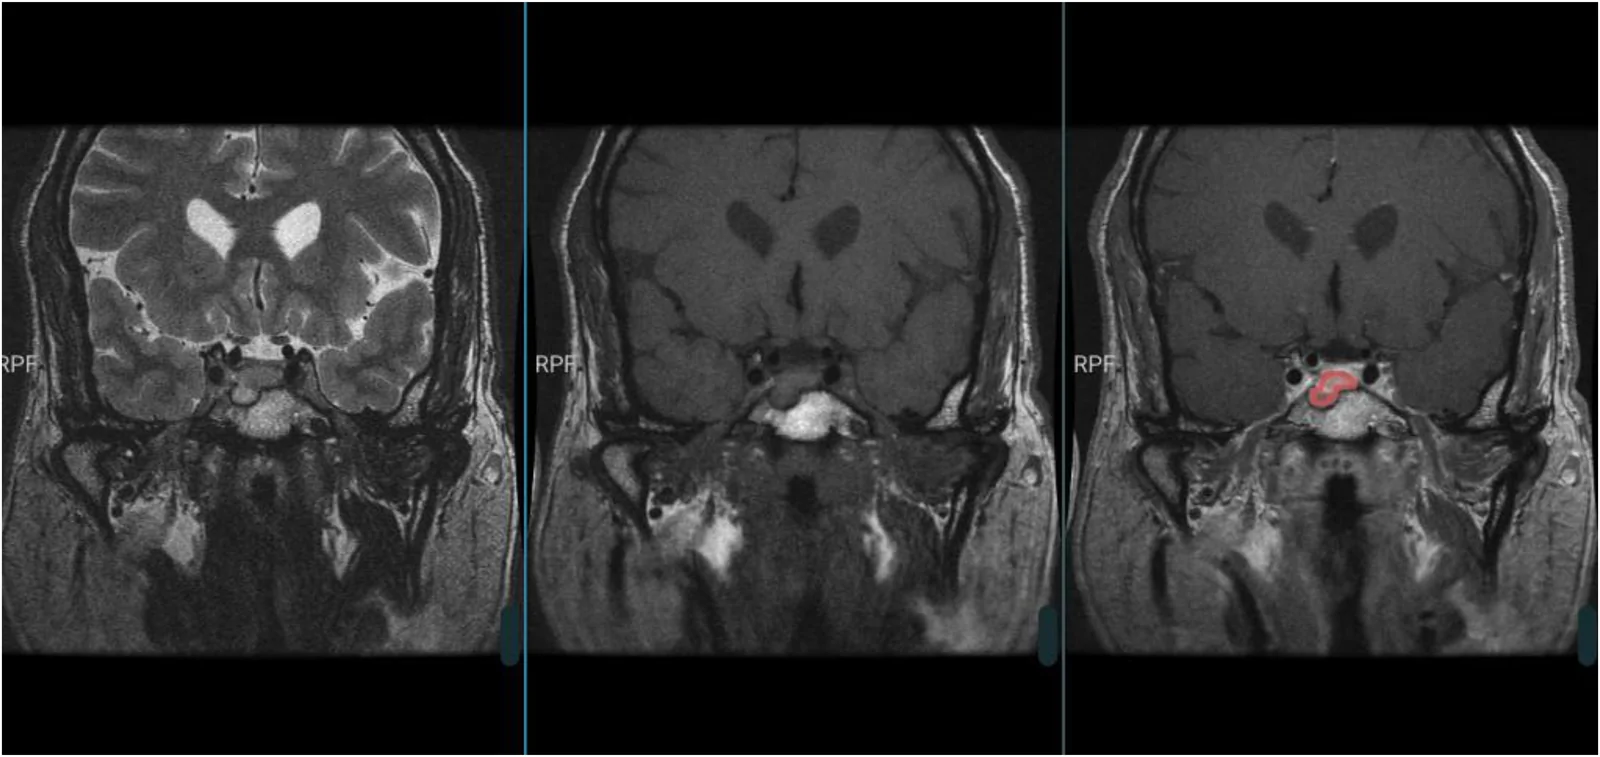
Magnetic resonance imaging of patient 3 showing a Knosp 2 invasive pituitary adenoma to the right cavernous sinus with an isointense-hyperintense signal in the T2-weighted sequence. The tumor is also contacting the chiasm.
